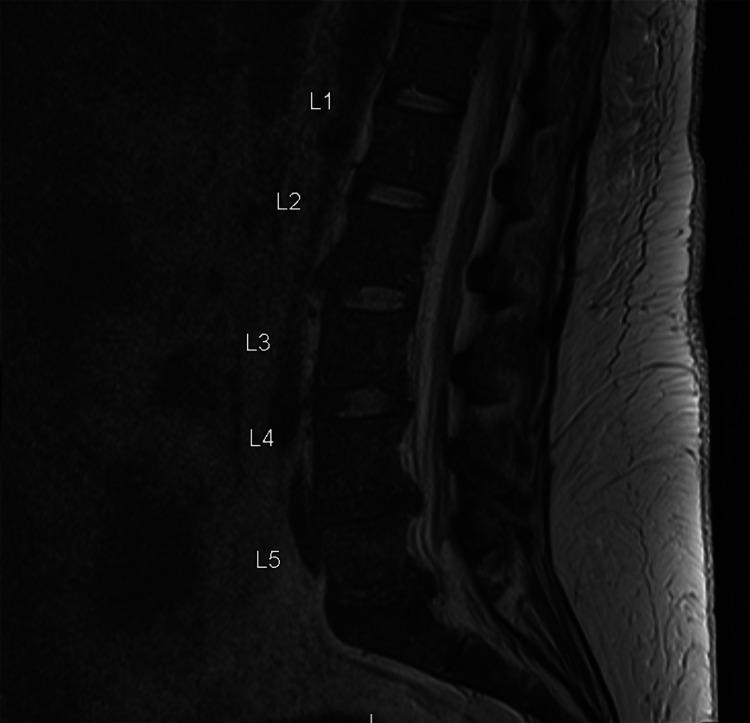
MRI lumbar spine: there are posterior protrusions at the L3-4 through L5-S1 levels. There is no spinal canal stenosis. There is mild to moderate left L5-S1 neural foramen narrowing.
